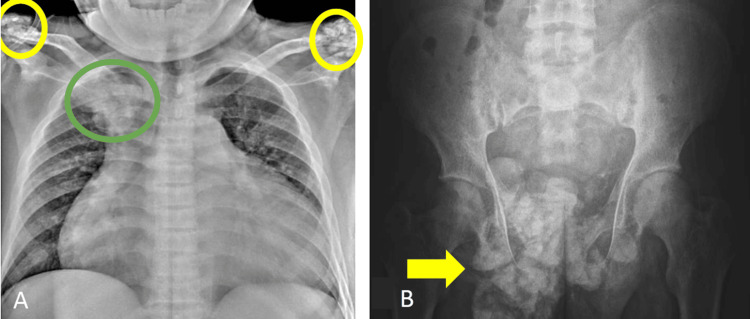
Radiograph of the chest and pelvis Chest radiograph (A) showing lobulated density projecting over the right upper hemithorax (green circle) measuring 5.2x6.4 (CCxW). Similarly, multifocal calcifications are observed in the bilateral acromioclavicular joint regions (yellow circles) measuring 4.5x4.8 cm (CCxW) in the right and 3.8x4.8 cm (CCxW) in the left. Pelvic radiograph (B) showing multilobulated calcifications seen in the right pelvic region (arrow) measuring 12.2x11.3 cm (CCxW).
